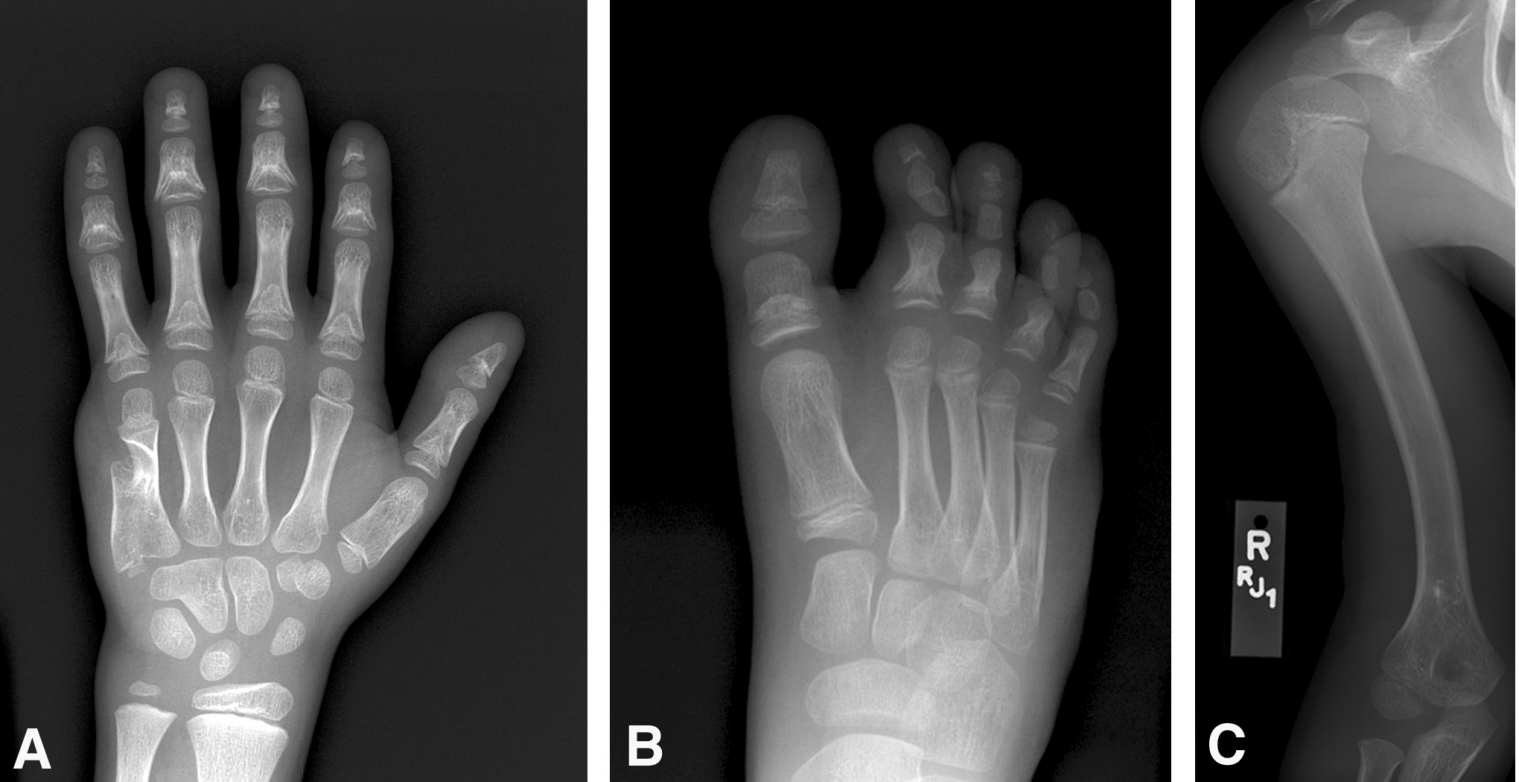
Radiographs of patient V-8. A) Residual appearance of postaxial polydactyly of the hand B) Abnormal appearance of some of the middle and distal phalanges of toes C) Mild lateral bowing of the humerus.
